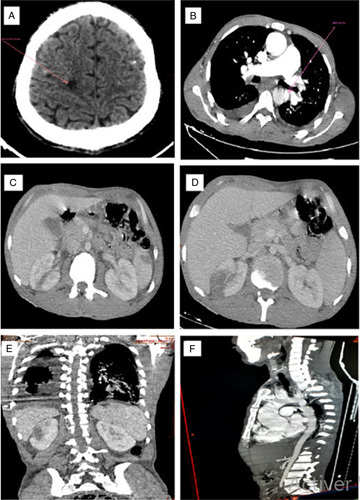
Brain computed tomography (CT) showing poorly limited hypodense right frontal brain spot (A), axial section thoracic CT showing thoracic aortic aneurysm (B), axial section abdominal CT showing splenic infarct (C), renal infarct (D), frontal section abdominal CT showing splenic and renal infarct (E), sagittal section, thoracic CT showing aortic coarctation (F).
